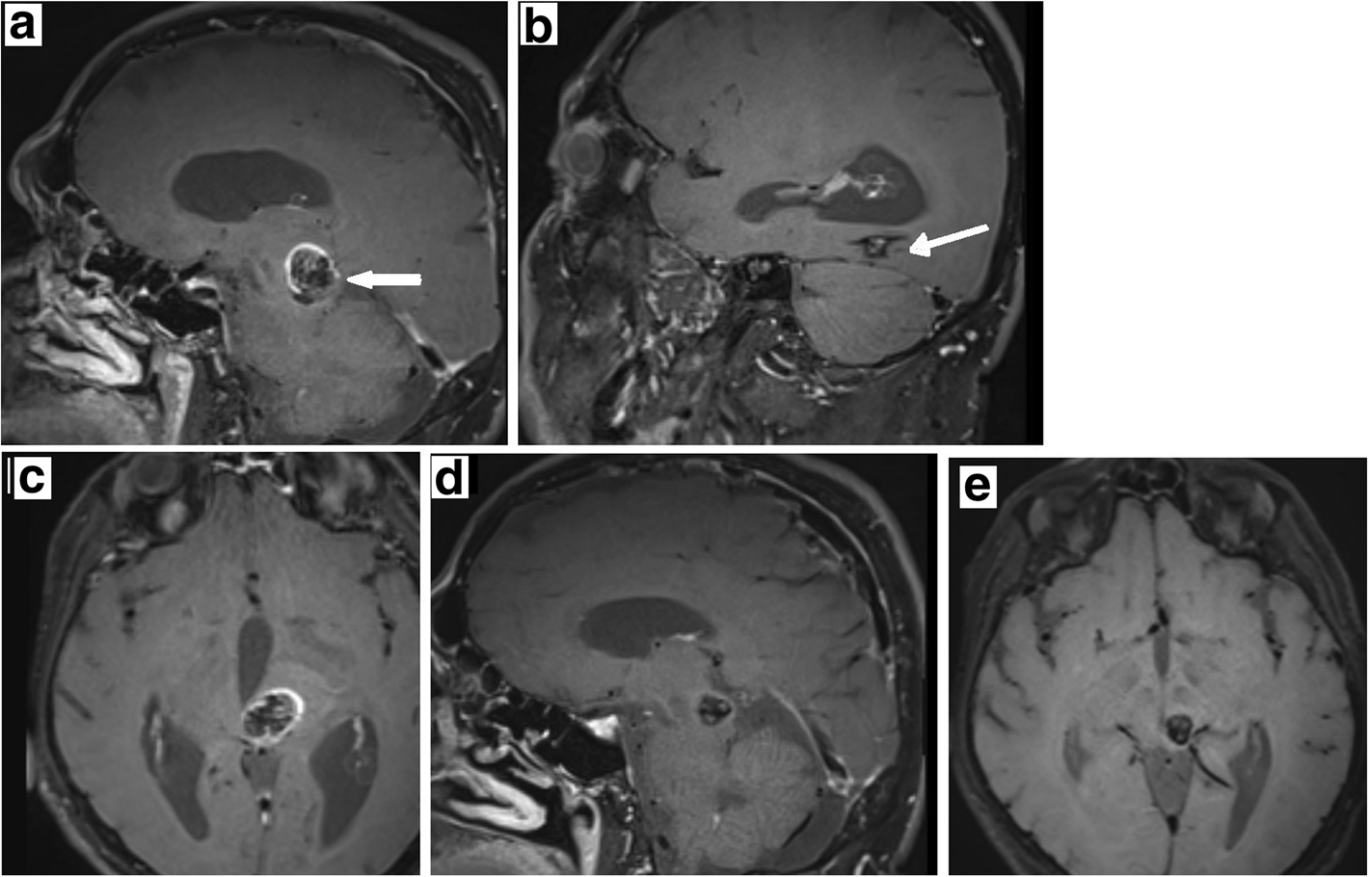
Intracerebral bleeding of a mesencephalic CCM, a Sagittal plane of a black blood MRI showing contrast enhancement of the mesencephalic CCM (arrow) (ruptured) (left). b Non bleeding second CCM localised in the occipital lobe (right) without contrast enhancement (arrow:CCM). c Same as in 1A. Black blood transverse plane of the mesencephalic CCM. d Sagittal section. Follow up black blood MRI 2 months after bleeding. The CCM blood is completely resorbed and no contrast enhancement can be seen. e Transverse section of D
